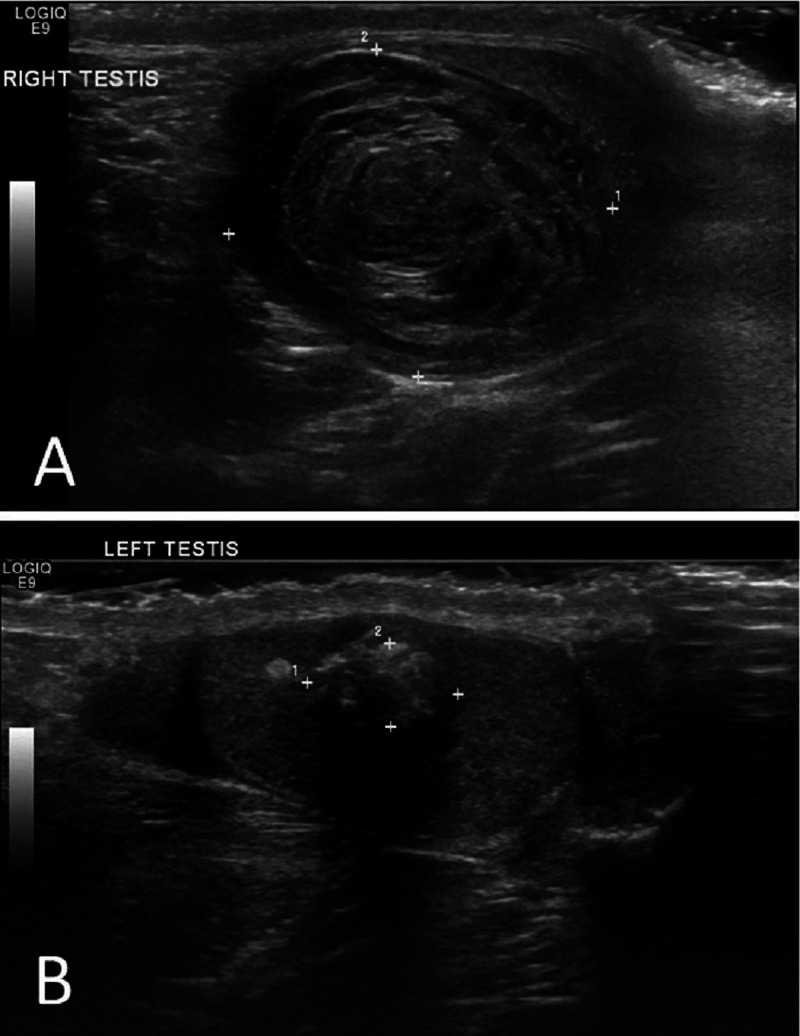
Ultrasound scan demonstrating bilateral testicular epidermoid cysts. (A) Right testicular cyst demonstrating the characteristic “onion ring” appearance. (B) Left testicular cyst demonstrating a mixed/alternating echogenic pattern.
